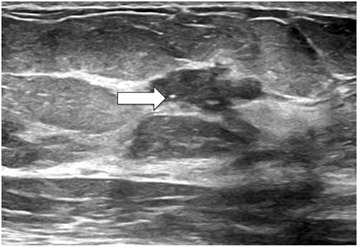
Ultrasound image of right breast mass where several small calcifications can be seen (*arrow*), representing an uncommon sonographic finding
